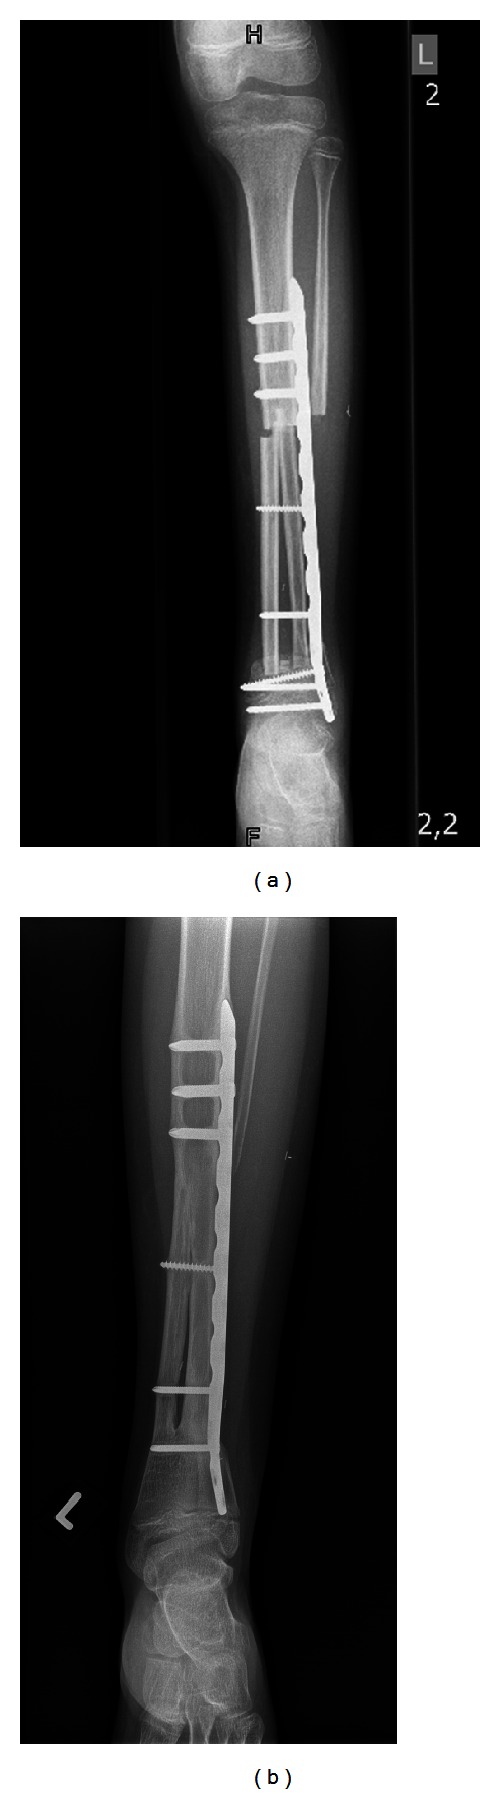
(Patient no. 7) (a) Radiograph of a 9-year-old girl with Ewing's sarcoma of the distal tibia diaphysis. Defect reconstruction (12 cm) was achieved by using bilateral fibular graft and plate osteosynthesis. Due to the small remaining distal epiphyseal fragment the screws had to be placed in the epiphysis. (b) Radiographic results 29 months after tumor resection giving good evidence of bony healing. The epiphyseal screws have been removed. Nevertheless the ankle shows a mild valgus deformity resulting in an MSTS score of 93%.
